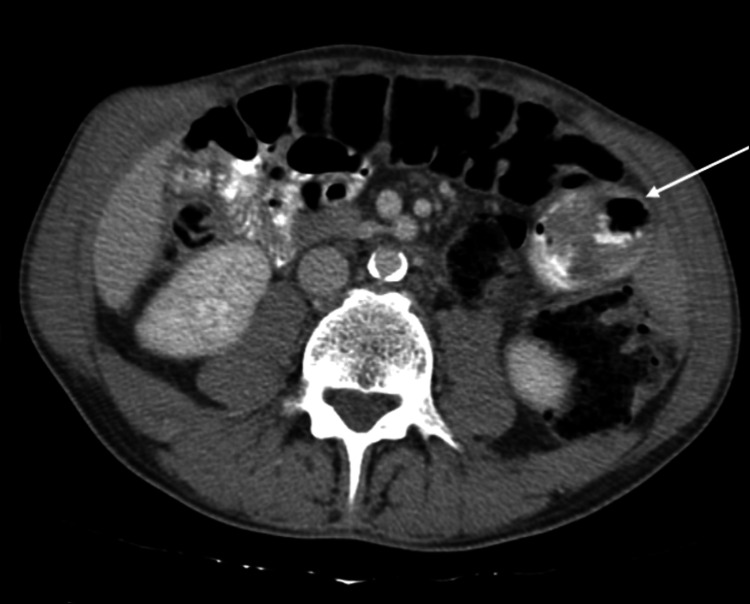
Abdominal CT demonstrating an endoluminal jejunal lesion, which was later confirmed to be jejunal adenocarcinoma (axial view)
